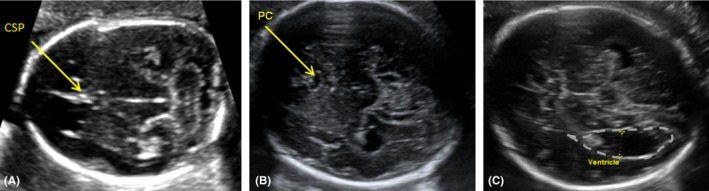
Axial views of head and brain using fetal ultrasound. A, At 18w3d gestation, an interhemispheric fluid collection is noted and was called a cavum septi pellucidi (CSP). No abnormal findings were documented. B, At 29w6d gestation, an interhemispheric fluid collection is again seen and now noted to have abnormal morphology consistent with a pseudocavum (PC). Additionally noted at this time (but not pictured) were teardrop‐shaped ventricles consistent with colpocephaly. C, At 31w2d gestation, the enlarged and tear‐dropped shaped bilateral lateral ventricles (left ventricle is depicted with dashed line) consistent with colpocephaly were again seen
